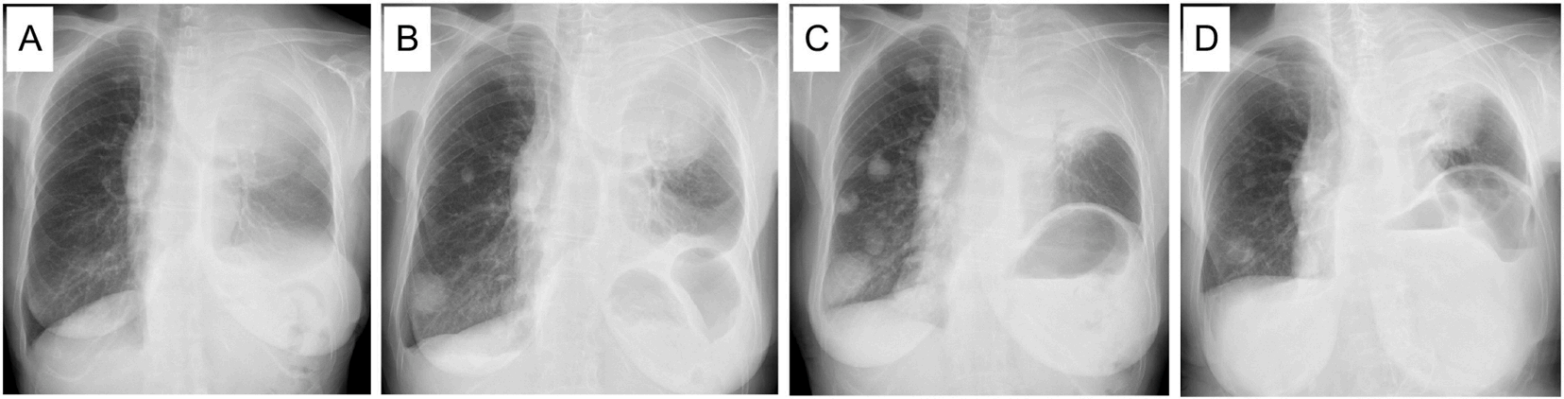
Chest X-ray findings. (A) A giant mass shadow was observed in the left upper lung field at the initial visit. (B) The pulmonary metastatic colonies in the right lung were obviously enlarged when second-line chemotherapy was introduced. F1CDx was performed at this time. (C) The lung metastatic colonies were further enlarged when osimertinib was introduced. (D) A marked anti-tumor response was observed after two months of osimertinib treatment.
